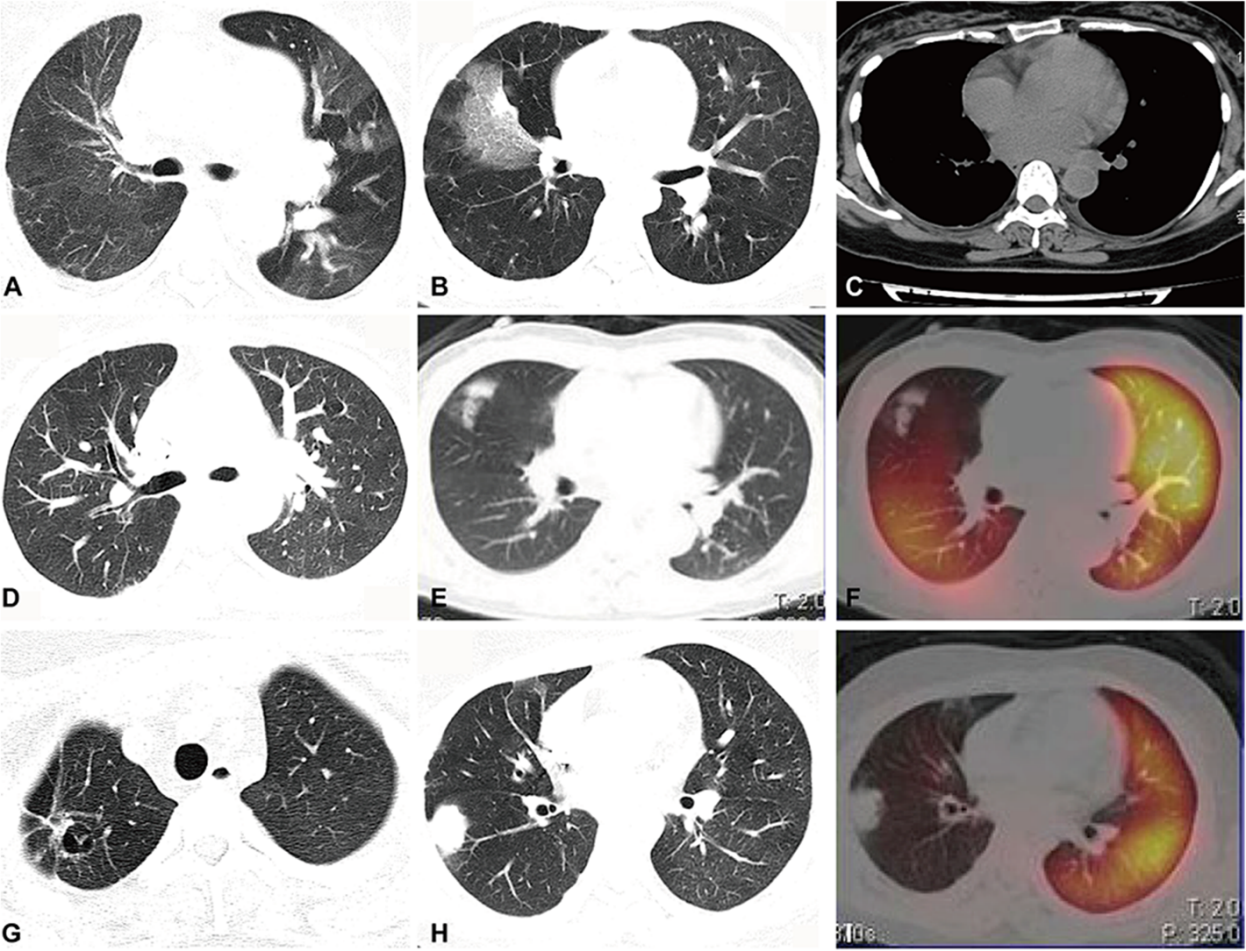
Pulmonary lesions on HRCT. a The mosaic sign in the left lung of a patient with stenosis of the left main PA. b Pulmonary infarction of the right middle lobe in a patient with severe stenosis of the right main PA. c Mild pleural effusion on the left side in a patient with pulmonary trunk dilation and pulmonary hypertension. d Bronchiectasis in the right lung in a patient with stenosis of the right main PA and its distant branches. Ground-glass opacity (e) in the right upper lobe of a TA patient with right upper pulmonary arterial branches involvement (f). Cavitation (g) and mass-like consolidation (h) in the patient with severe stenosis of the right main PA (i)
